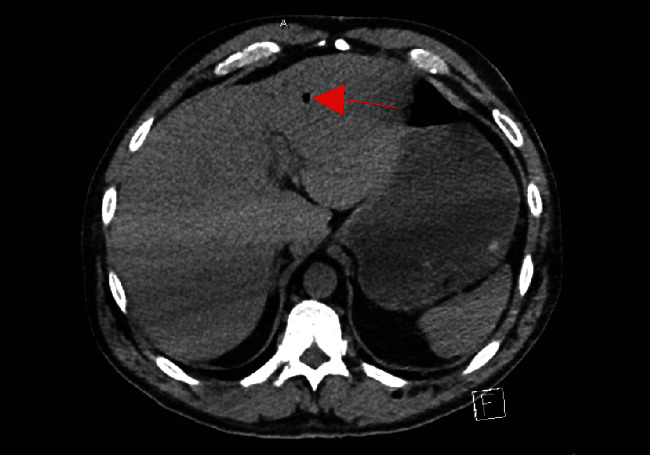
Noncontrast computed tomography with evidence of portal venous gas.
